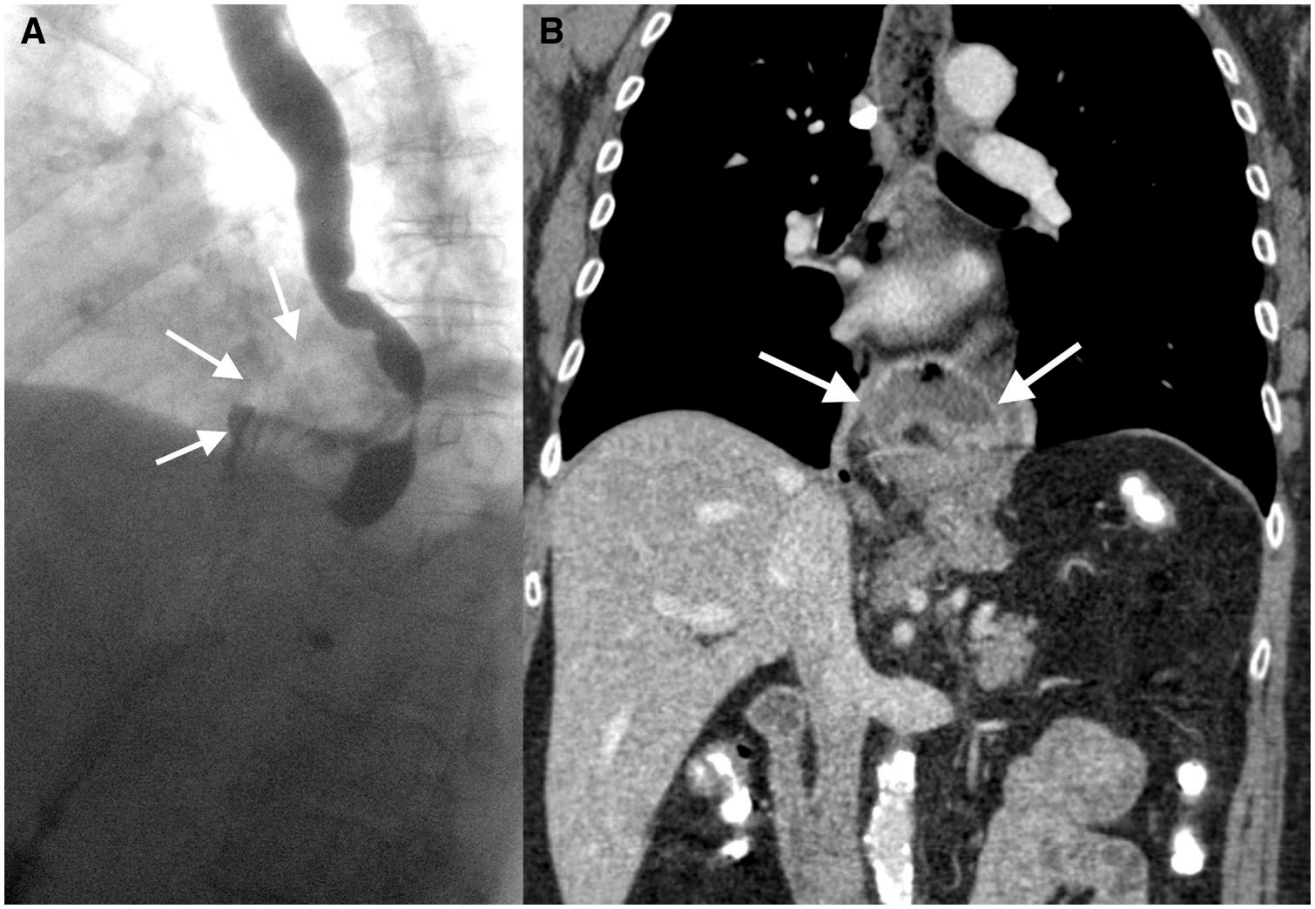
Supradiaphragmatic migration of a slipped stomach. (A) One day after Nissen fundoplication, fluoroscopy revealed paraoesophageal slipping of gastric parts into the supradiaphragmatic region (arrow). (B) CT confirmed paraoesophageal slipping of the fundoplication wrap (arrows).
